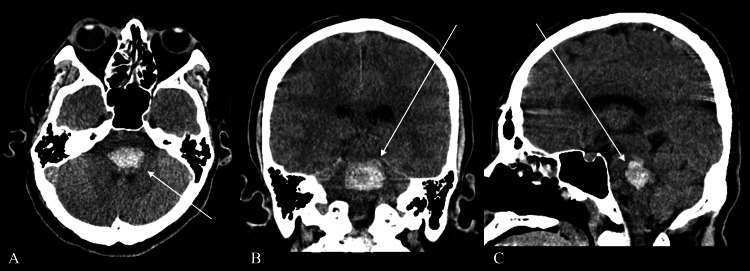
CT brain images CT: Computed Tomography (A) Axial, (B) Coronal, and (C) Sagittal views, demonstrating a hyperdense acute intracerebral hematoma centered in the pons with mass effect but no upstream hydrocephalus
